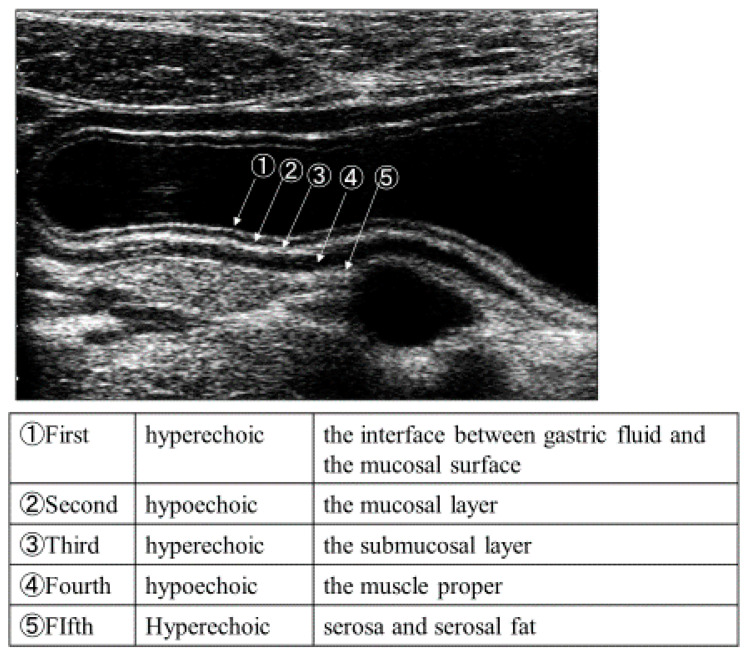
Five-layer stratification of stomach by sonography. The first inner hyperechoic layer represents the interface between gastric fluid and the mucosal surface; the second hypoechoic layer shows the mucosal layer; the third hyperechoic layer shows the submucosal layer; the fourth hypoechoic layer shows the muscle proper; and the fifth hyperechoic layer shows serosa and serosal fat.
